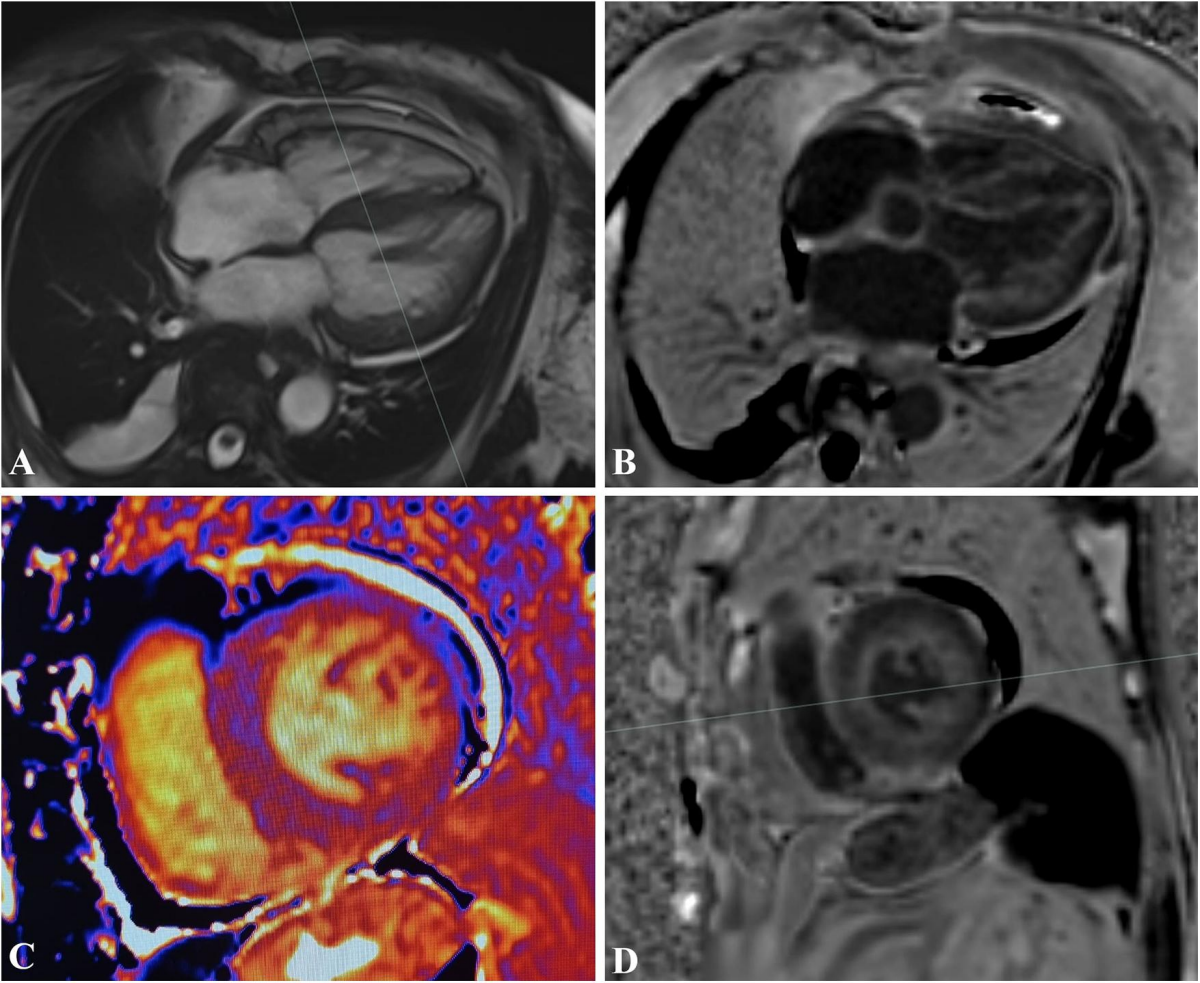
61-year-old patient with cardiac amyloidosis. Four-chamber balanced-steady state free precession (b-SSFP) MRI (A) shows thickening of the left ventricle. PSIR sequence highlights circumferential subendocardial LGE of left and right ventricular walls in four-chamber (B) and short-axis (D) T1 native Mapping (D) demonstrates increased intramyocardial value in ventricular walls.
